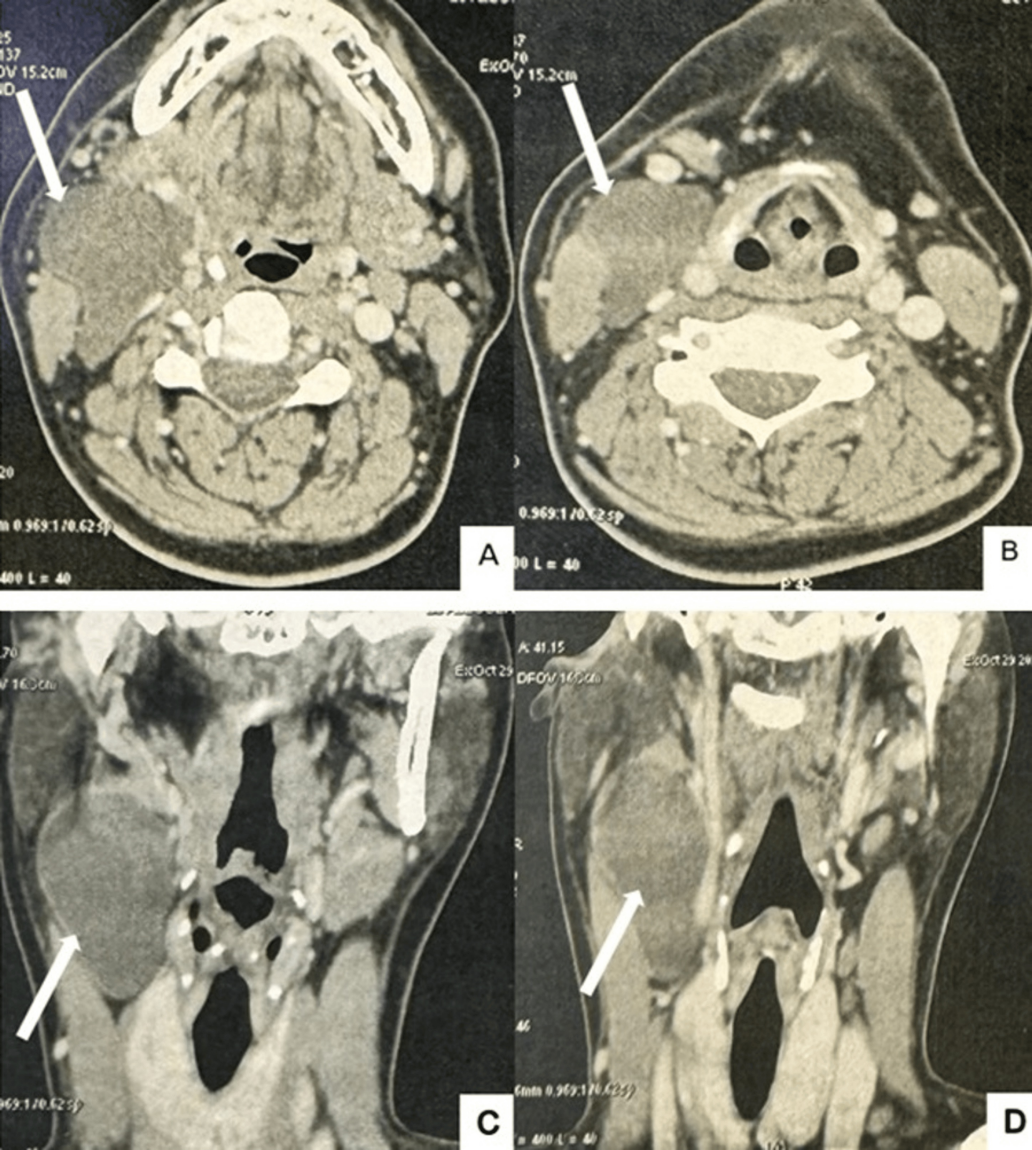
Contrast-enhanced cervical CT scan demonstrating a well-defined cystic lesion in the right lateral cervical region displacing adjacent structures without signs of invasion (arrows). (A, B) Axial views. (C, D) Coronal views.
